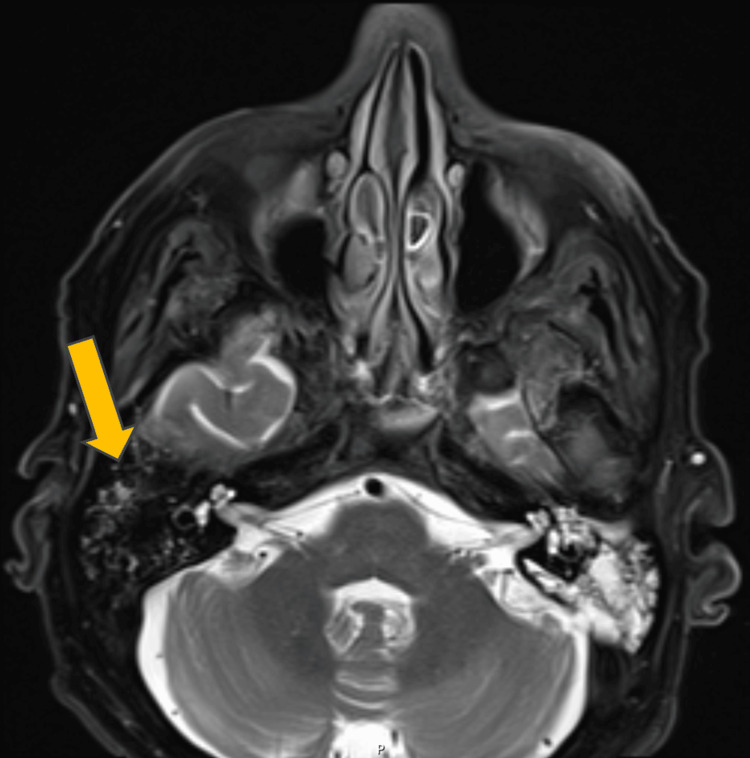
MRI of the brain, axial section, T2, fat saturation, showing small right mastoid effusion in addition to the opacification of left mastoid air cells.
